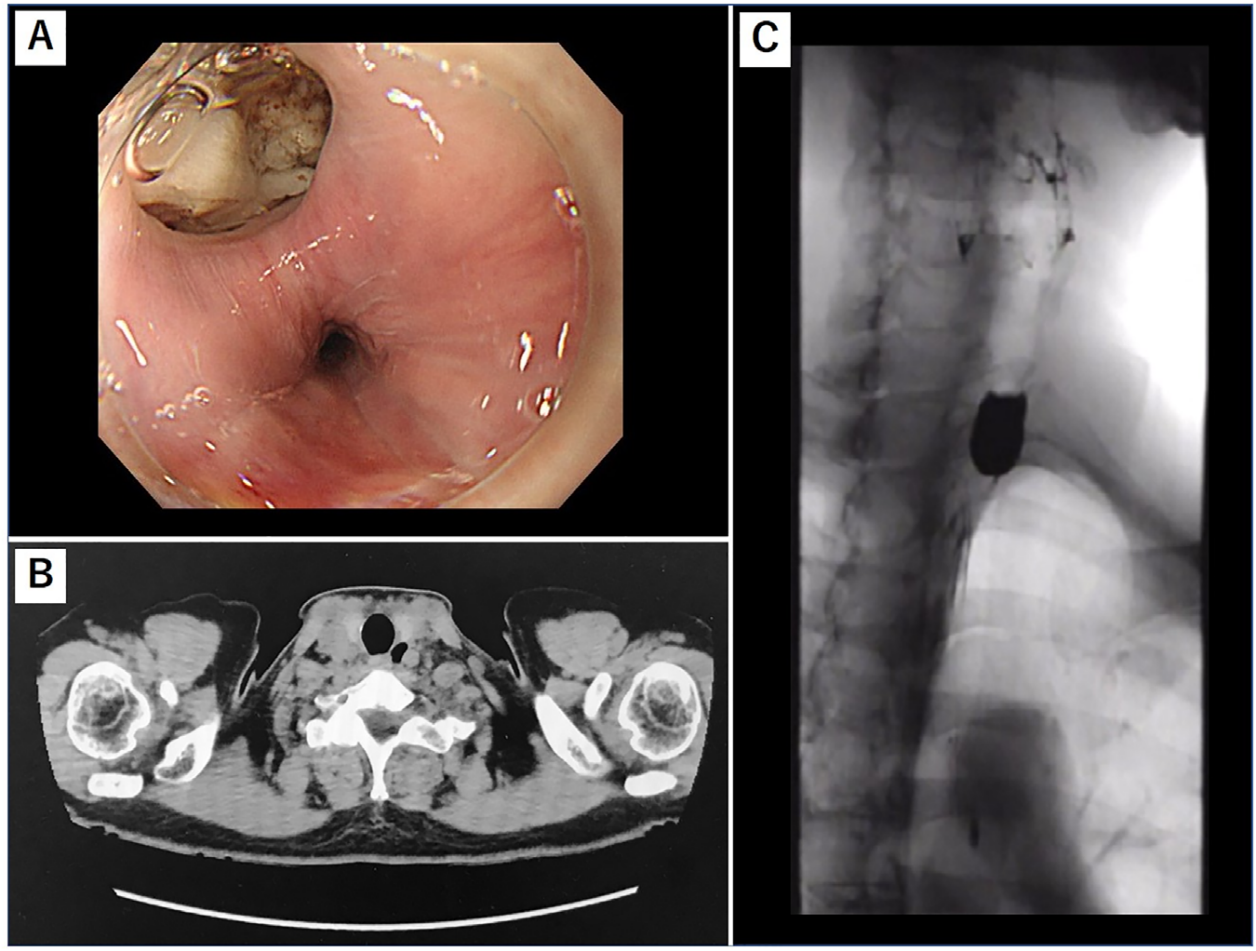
(A) Endoscopic image of Killian–Jamieson diverticulum. Food impaction is seen at 11 o'clock. (B) CT image of Killian–Jamieson diverticulum. The diverticulum lies off midline inferior to the cricopharyngeus muscle and located at the anterolateral wall of the cervical esophagus. (C) Barium esophagram revealing pooling of barium in the diverticulum
